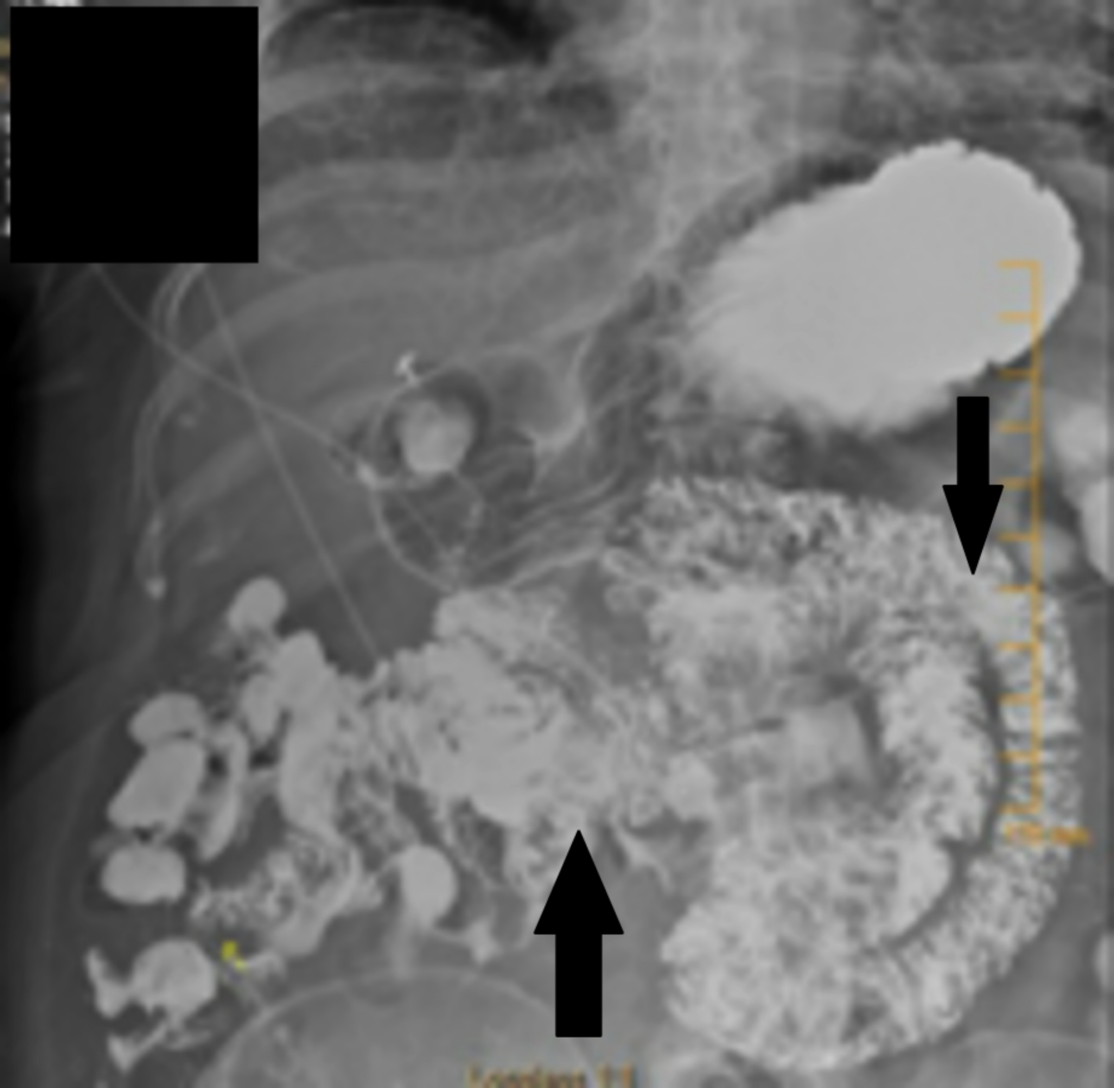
X-ray barium small bowel follow-through with arrows showing opacified and non-opacified small bowel loops
